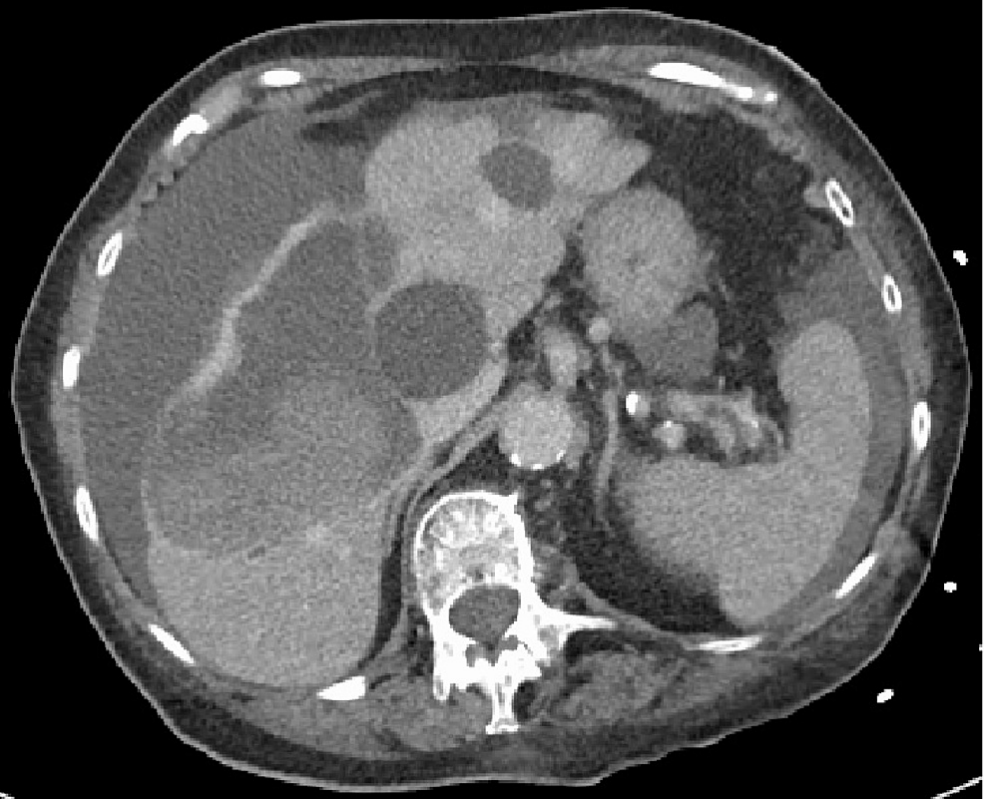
CT abdomen showing moderate hemoperitoneum along with multiple bleeding spots in the liver
